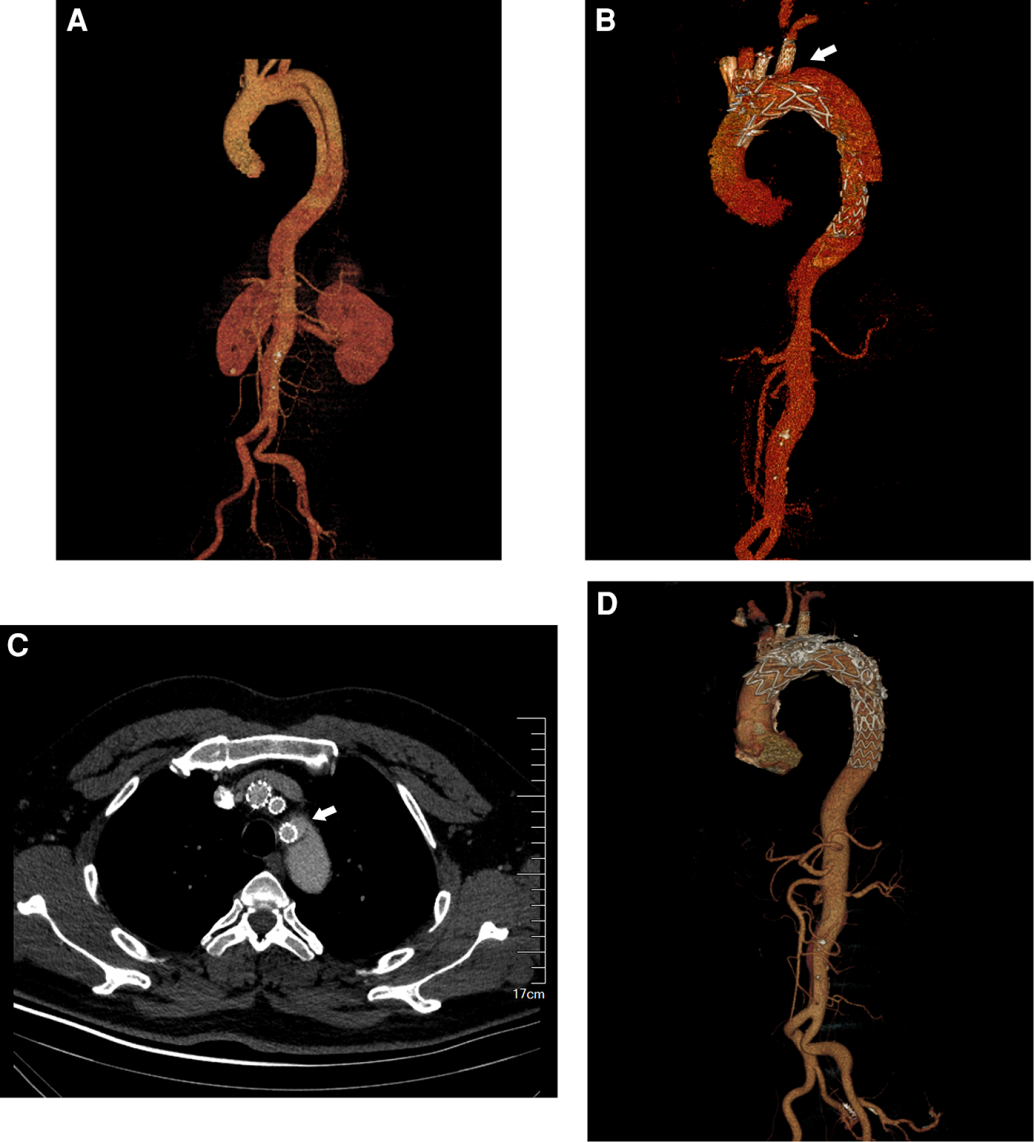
A 45-year-old male patient underwent fb-arch repair for chronic aortic dissection. The patient developed type IIIc endoleak around the left subclavian artery during the follow-up. (A) The CTA imaging of the aortic arch involved by dissection. (B) The CTA imaging of the Type IIIc endoleak near the left subclavian artery after fb-arch repair. (arrow). (C) The cross-sectional image of the Type IIIc endoleak (arrow). (D). After two false lumen coil embolization, the original endoleak disappeared and the aorta was well remodeled.
